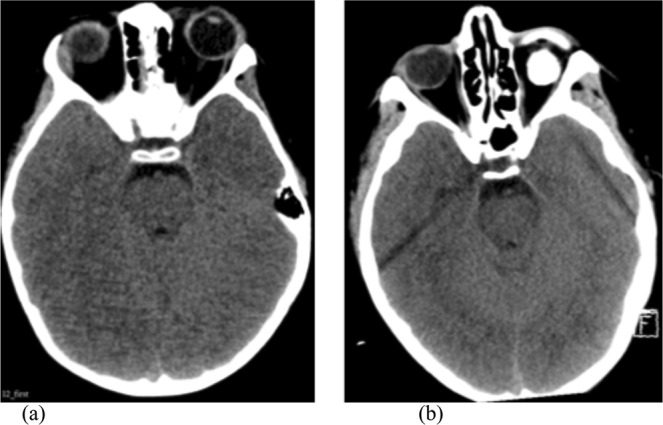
Representative CT images of basal cistern appearance. Patient with a corrected basal cistern volume ratios of: (a) 0.0054 [just below threshold], ICP = 12 mmHg (b) 0.0058 [just above threshold], ICP = 25 mmHg. Both demonstrate patent suprasellar cisterns.
